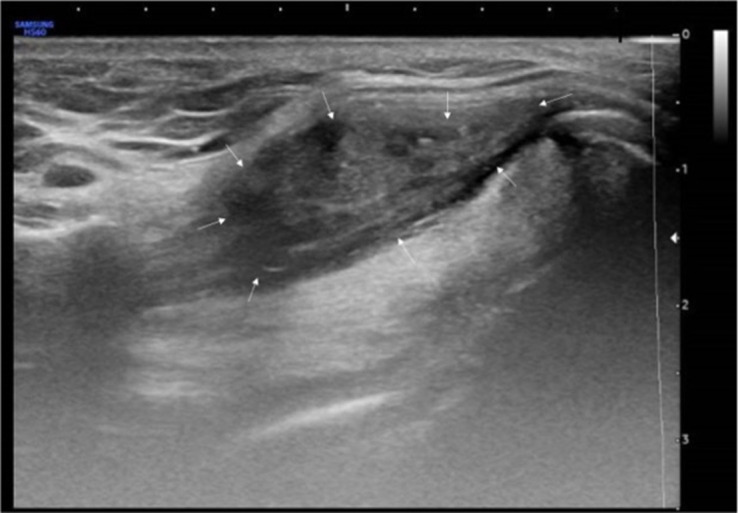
Neck ultrasonography 4 months after ethanol ablation; cystic component almost completely resolved.
